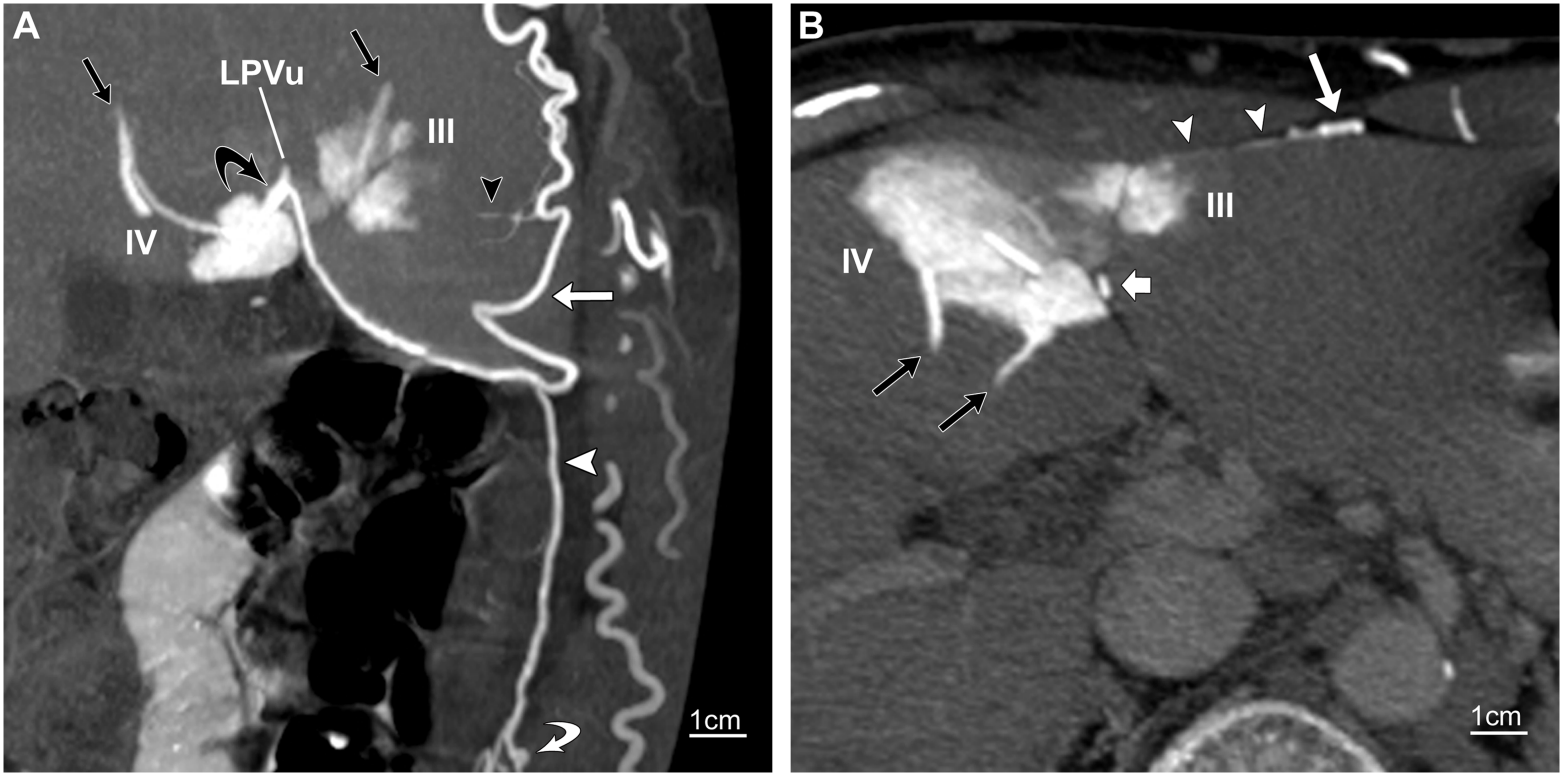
Inferior and superior paraumbilical veins. CT venogram of chest and abdomen in a 53-year-old man with SVC obstruction. (A) Oblique sagittal 10-mm maximum-intensity-projection (MIP) through the umbilical fissure shows an inferior paraumbilical channel (white arrowhead) ascending from deep epigastric veins (curved white arrow). It receives an ensiform vein (straight white arrow) and terminates in the left portal vein (LPVu). Note antegrade filling of a second-order portal branch (curved black arrow) and its territory in segment IV. Also shown are tiny superior paraumbilical veins destined for segment III, which form a tiny venous arch (black arrowhead) and are supplied by the ensiform vein. Straight black arrows point to hepatic veins. (B) Slightly oblique transverse 7-mm MIP shows the collateral channel (short white arrow) in the umbilical fissure and the opacification of segment IV. The tiny superior veins (arrowheads) opacify segment III. The long white arrow points to the ensiform vein; black arrows indicate hepatic veins.
